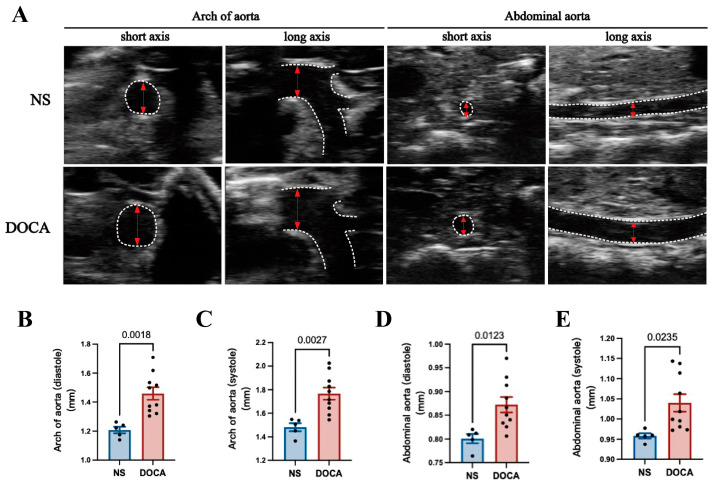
Ultrasound imaging and quantification of aortic remodeling in DOCA salt-sensitive hypertension. (A) Representative ultrasound images of the aortic arch and abdominal aorta during systole in both short and long axis views. (B,C) Comparison of the inner diameter (red arrows) of the aortic arch between the NS and DOCA groups during both (B) diastole and (C) systole. (D,E) Comparison of the inner diameter of the abdominal aorta between the NS and DOCA groups during both (D) diastole and (E) systole.
